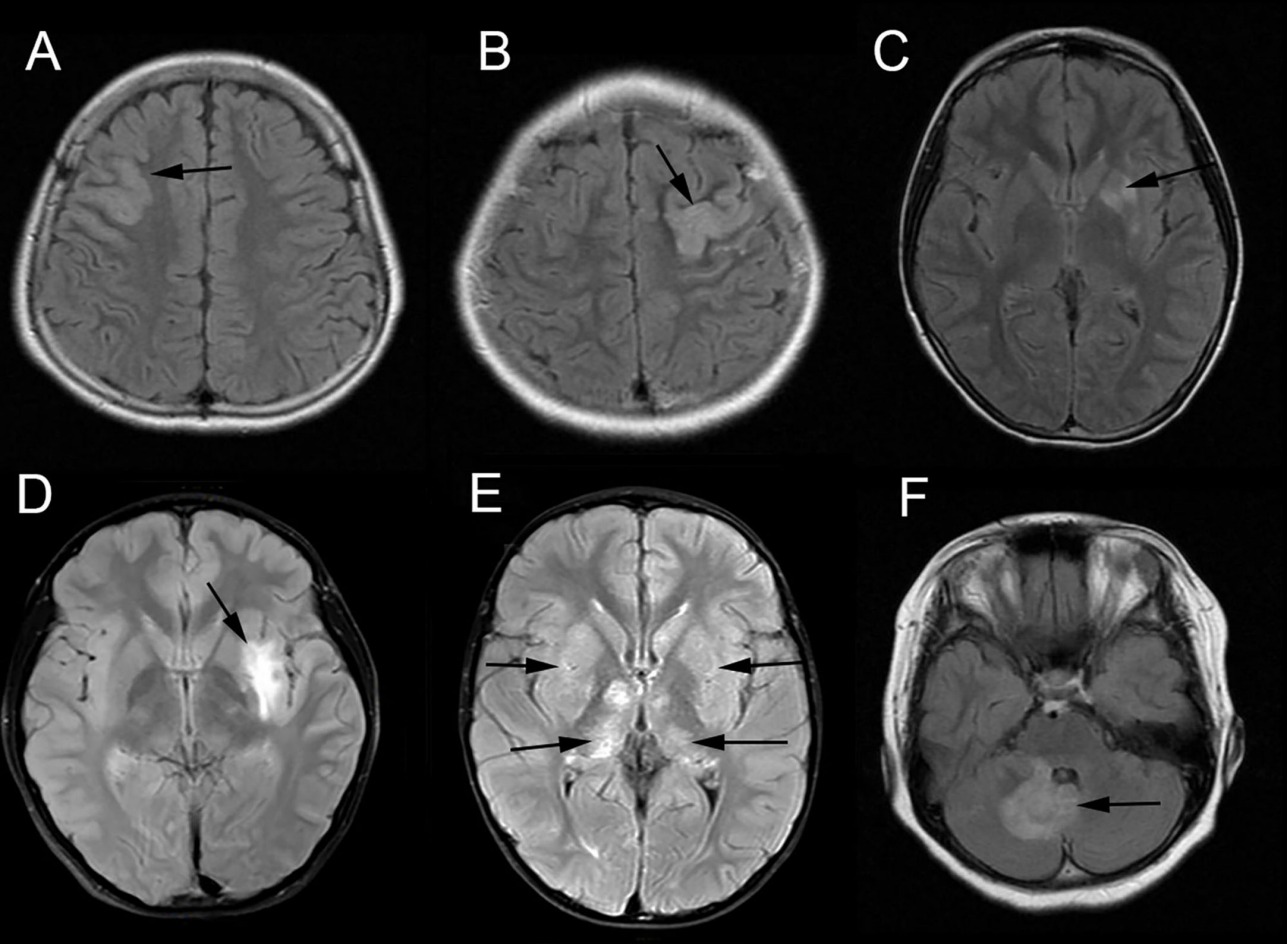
Brain magnetic resonance imaging (MRI) of patients having MOG antibody-positive pediatric autoimmune encephalitis without demyelination. The abnormal hyperintensity lesions on axial T2-weighted fluid-attenuated inversion recovery (T2-FLAIR) image were observed in the right frontal cortex (A, Patient 17), left parietal cortex (B, Patient 18), left basal ganglia (C, Patient 13), left insular cortex and basal ganglia (D, Patient 16), bilateral basal ganglia and thalamus (E, Patient 8) and right cerebellum (F, Patient 18).
